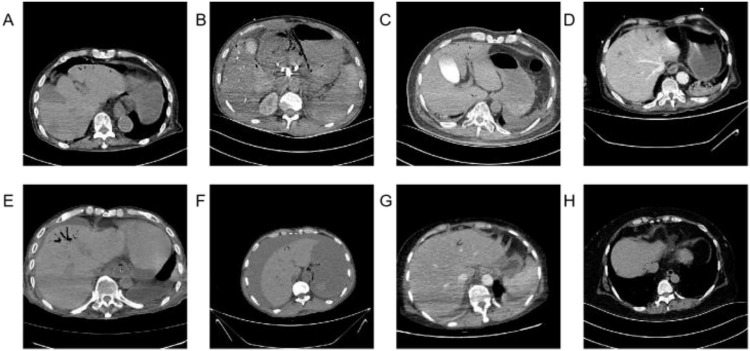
Representative contrast-enhanced CT images of HPVG confined to a single hepatic segment (A–H). HPVG appears as peripheral branching/punctate hypoattenuation along portal venous branches.
